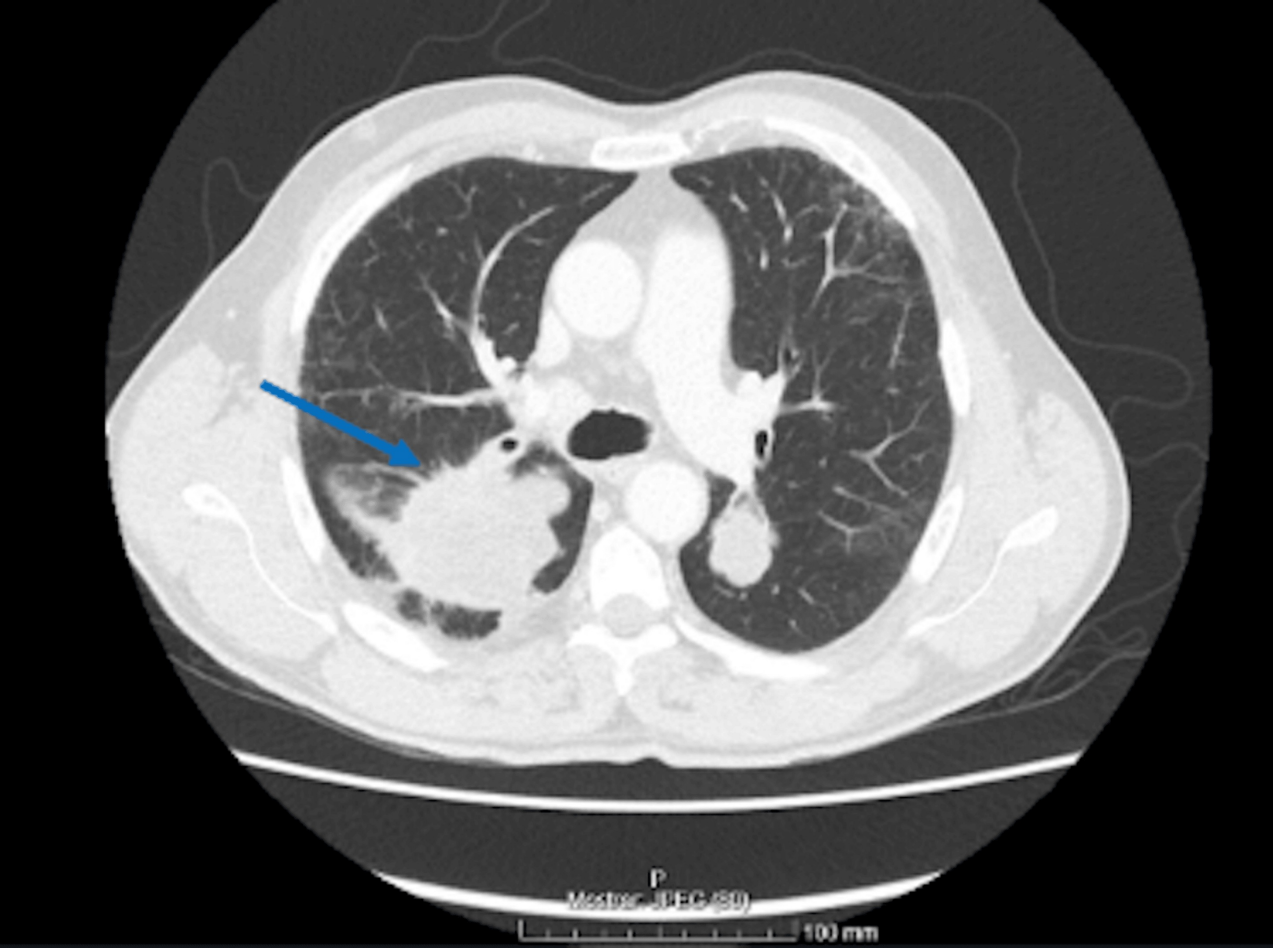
Abdominal, pelvic, and thoracic CT showing a mass in the right lung measuring 76 × 67 × 63 mm in the posterior segment of the upper lung lobe
